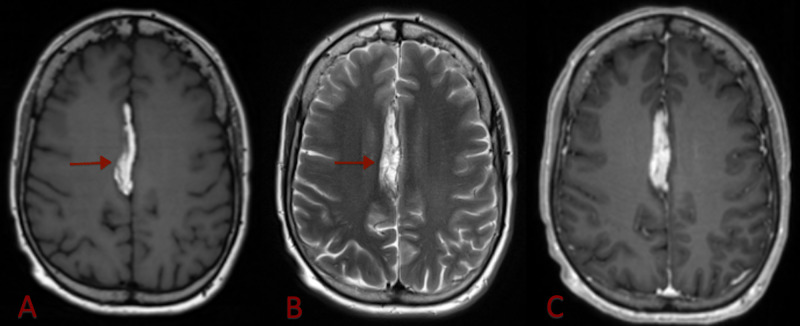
Axial magnetic resonance images of the brain Axial magnetic resonance images of the brain show a midline hyperintense lesion (red arrow) on both T1 pre-contrast (A) and T2 (B) weighted images. There is a lack of enhancement on T1 post-contrast image (C). These findings are consistent with fat.
